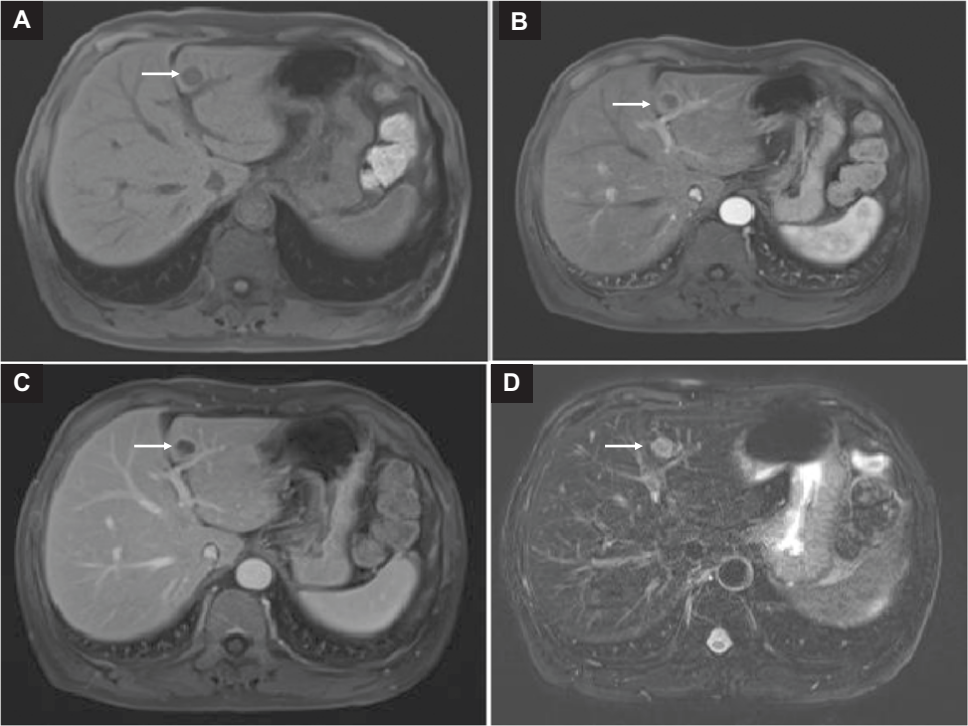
Liver metastasis (white arrow) of preoperative magnetic resonance images. a T1-w GE. b T1-w arterial enhanced. c T1-w venous enhanced. d T2-w
